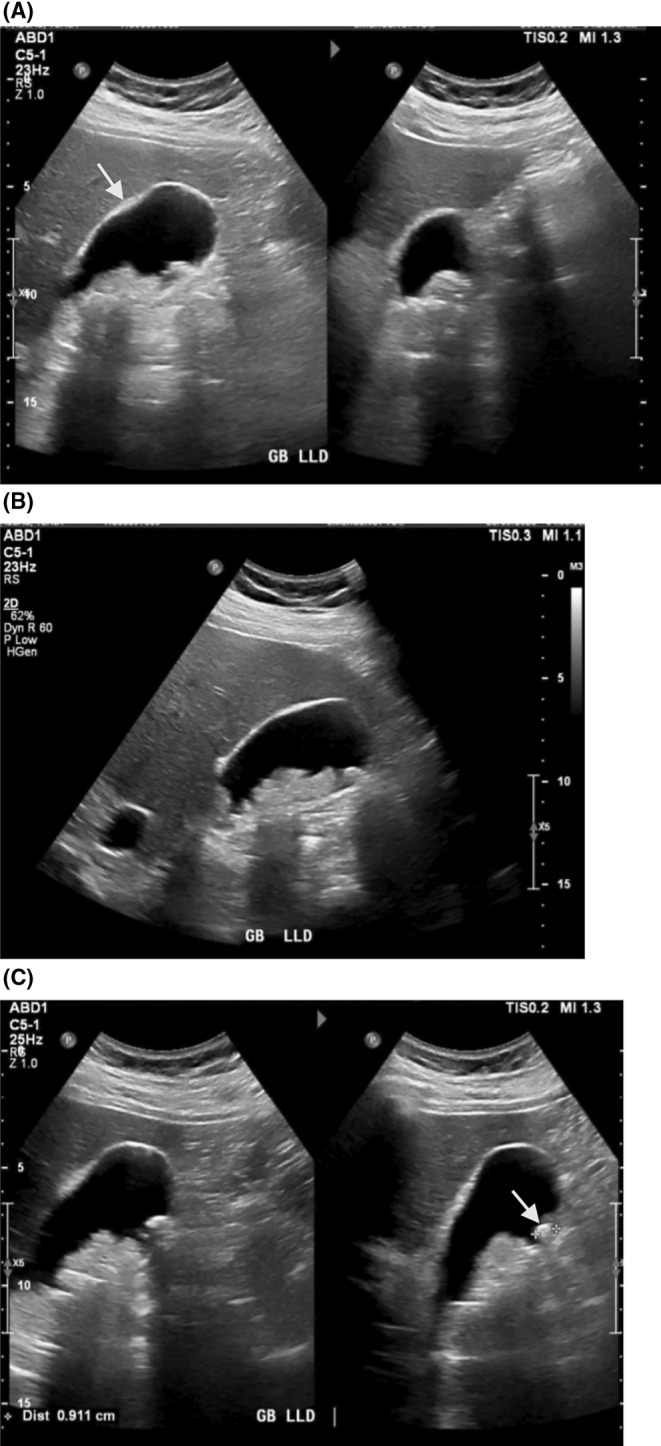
Ultrasound images showing a distended gall bladder (A). No pericholecystic fluid with mild wall thickening of 3.2 mm (arrow). Multiple calculi and internal sludge (B). The largest stone measuring 9 mm (arrow) (C).
